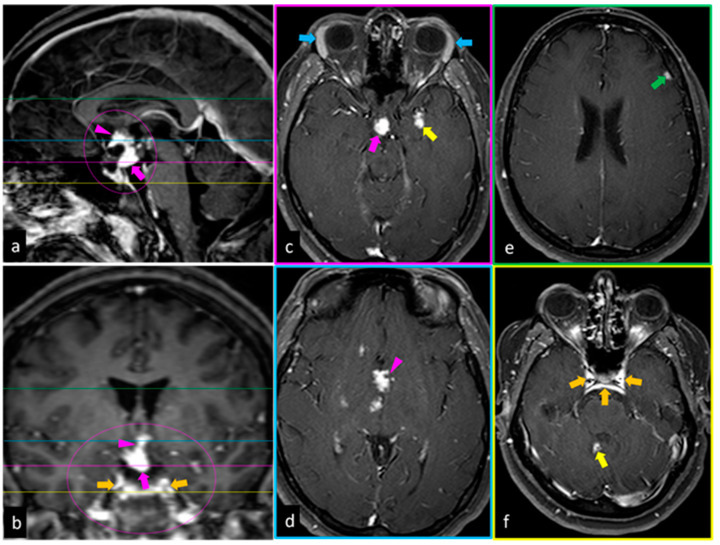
Ref. [53] A case of NS. Sagittal (a), coronal (b), and axial (c,d) post-contrast T1-weighted MR images show an extensive enhancement of the pituitary gland (pink arrows) and stalk (pink arrowheads), which is markedly enlarged. Both diffuse and nodular patterns are evident. A small extra-axial enhancing mass of dura is found (green arrow in (e)). Coronal (b) and axial (f) post-contrast T1-weighted MR images show the widespread thickening and enhancement of the leptomeninges (orange arrows) along the convexities of the brain, near the basal cisterns. Contrast-enhanced T1-weighted axial MR image shows enhancing parenchymal mass lesions in the left temporal lobe (yellow arrow in (c)) and in the cerebellum (yellow arrow in (f)), and bilateral enlarged lacrimal glands (light blue arrows in (c)).
